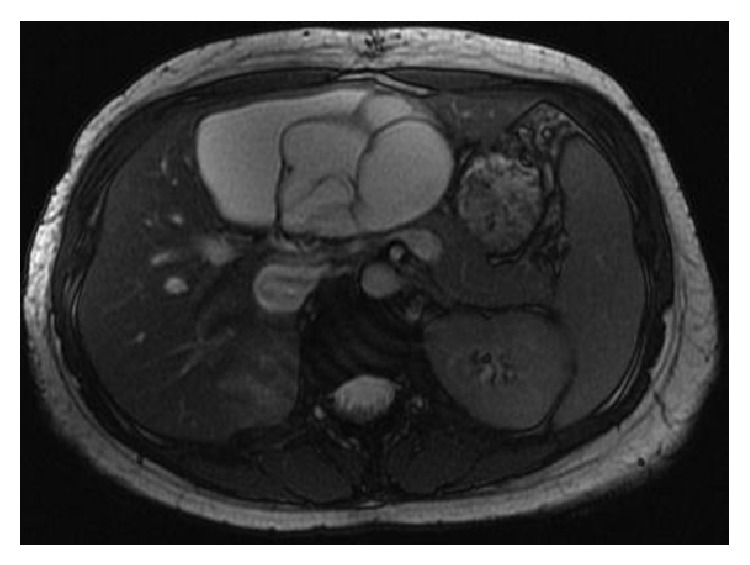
Name: axial 2D FIESTA. Description: multiple low T2 signal septations were seen within the mass on MRI.
